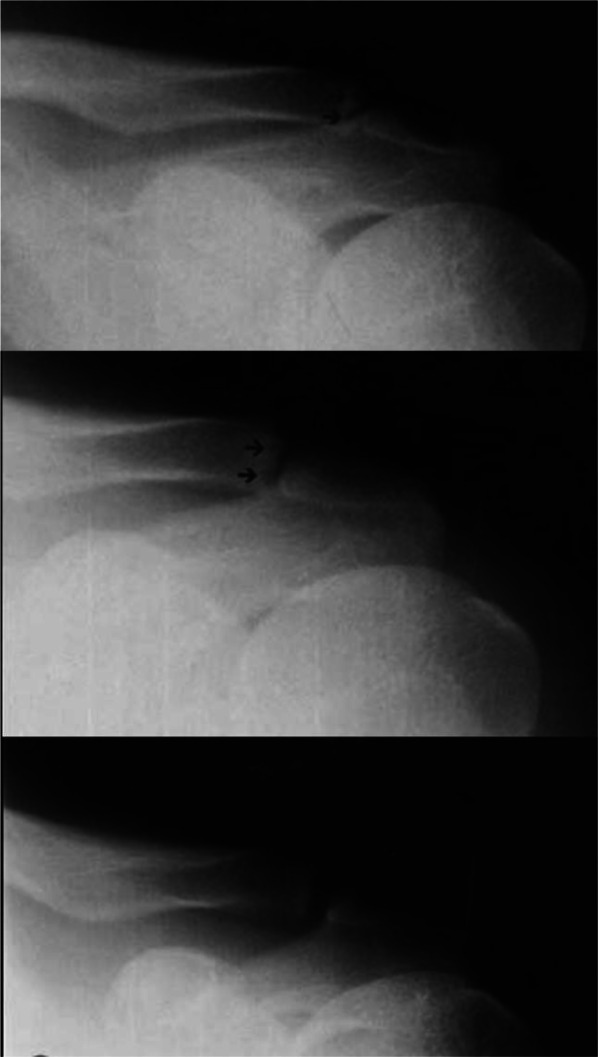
Clavicle X-rays. Top: cystic erosions noted at distal clavicle (arrows). Middle: six months later demonstrating decreased acromioclavicular joint space and reorganization of distal clavicle (arrows). Bottom: One year after initial visit, showing normal distal clavicle. Reproduced from Mestan et al. [47], with permission
